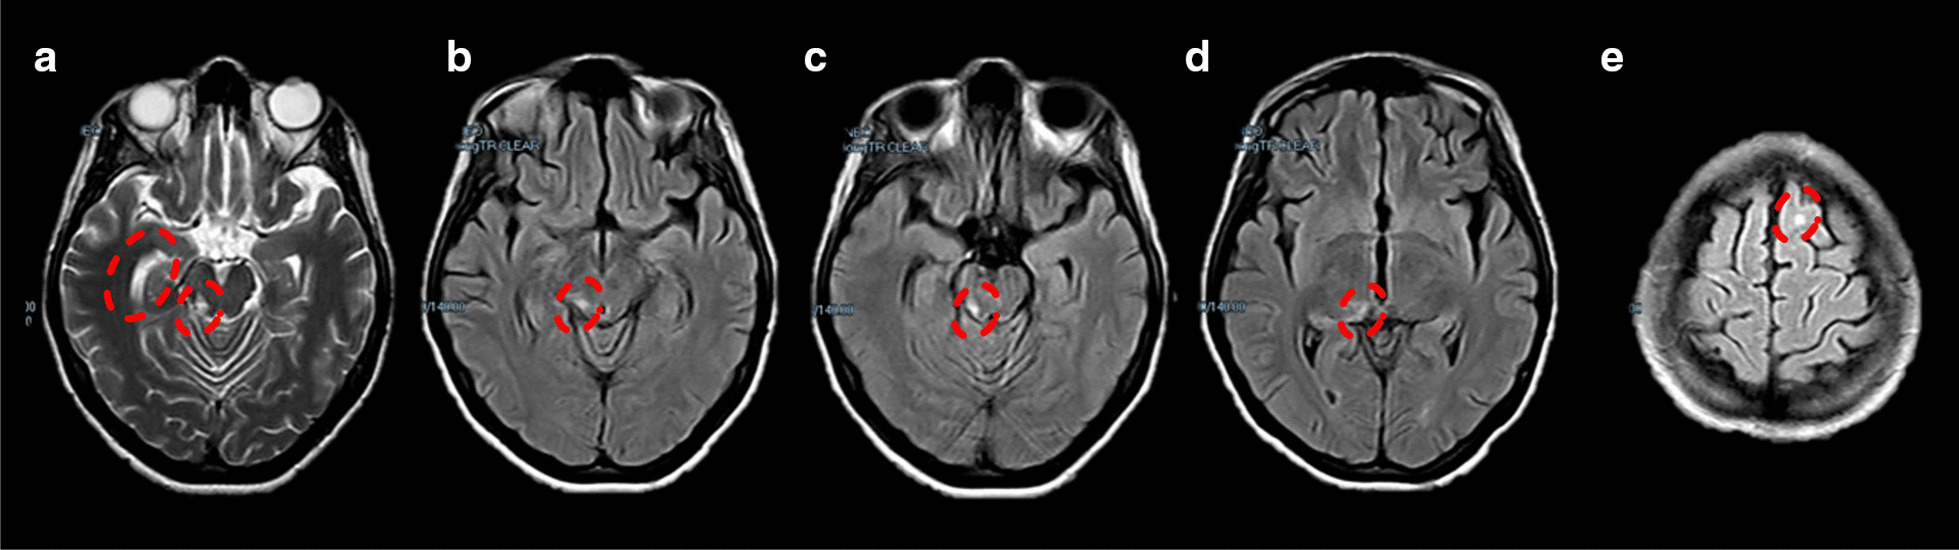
Brain magnetic resonance imaging findings in a patient with diffuse axonal injury. Axial T2-weighted (a) and fluid-attenuated inversion recovery magnetic resonance images of the brain (b–e) showing multifocal hyperintensities (red circles) located at the gray-white matter interface compatible with diffuse axonal injury of grade III
